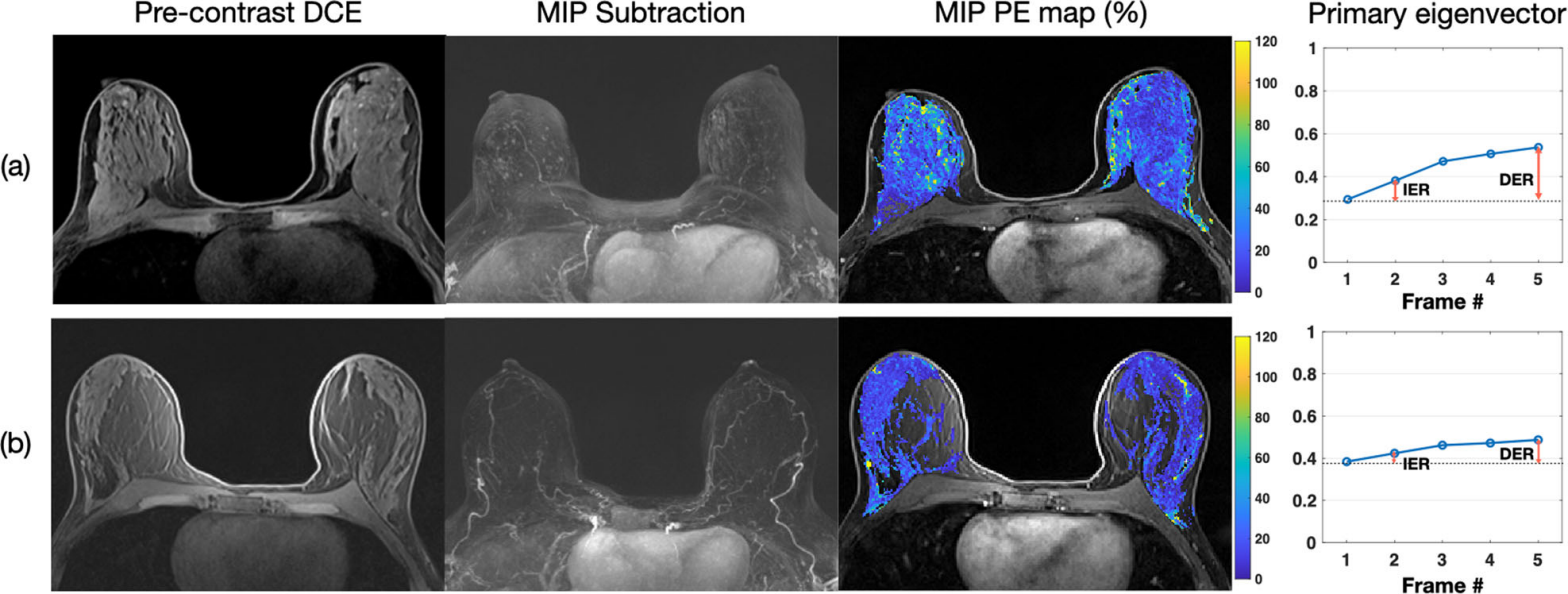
MRIs and BPE of two representative dense-breast normal-weight postmenopausal women with (a) high-risk non-*BRCA* mutation and (b) nonhigh-risk. MRI images include precontrast DCE-MRI, MIP of first postcontrast subtraction DCE-MRI, MIP of ten slices of PE map, and the primary eigenvector used to measure IER and DER. a A 49-year-old woman with a lifetime risk of 45.0% and a BMI of 22.45. FGT level is extreme fibroglandular tissue, and BPE level is marked. b A 53-year-old woman with a lifetime risk of 7.7% and a BMI of 20.08. FGT level is heterogeneously fibroglandular tissue, and BPE level is minimal
